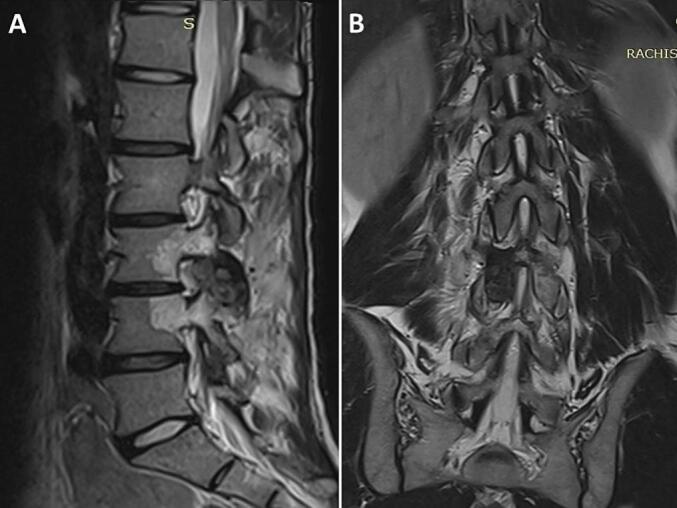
(A, B): The MRI depicted edematous signal abnormalities in the right lateral and posterior arches of the L3 and L4 vertebrae, characterized by hyperintensity on T2 and STIR sequences and hypointensity on T1. Post Gadolinium injection, these signals exhibited homogeneous enhancement. The abnormalities were centered on the ipsilateral posterior zygapophyseal joints, notably enhancing the right L4 zygapophyseal joint space, and extended into the right lumbar psoas and paravertebral muscles, without evident collections.
